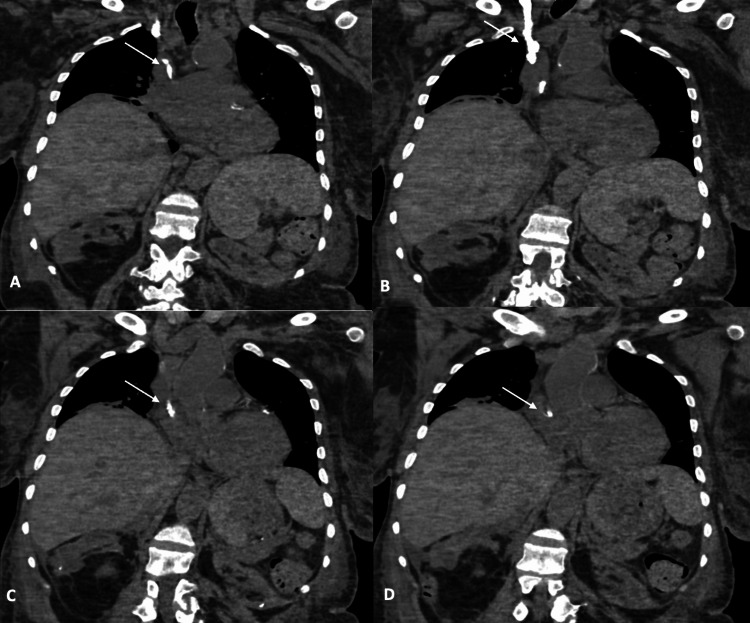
Non-contrast coronal CT images from anterior to posterior (A-D). Images show an irregular, linear, cylindrical radio-opaque density in the right internal jugular vein.
